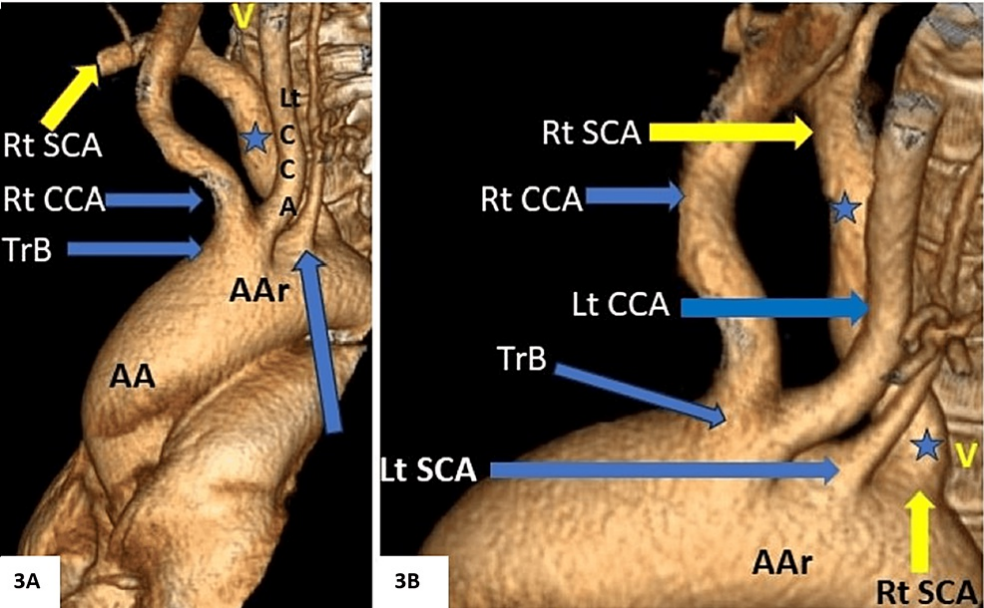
3D volume rendered images from a CT angiography study (A and B) showing aberrant right subclavian artery (Blue Star, annotated) arising as the last branch of the aortic arch, distal and posterior to the left subclavian artery, and traversing to the right side in the prevertebral course AA: ascending aorta; AAr: aortic arch; TrB: truncus bicaroticus; CCA: common carotid artery; SCA: subclavian artery; V: vertebral column
